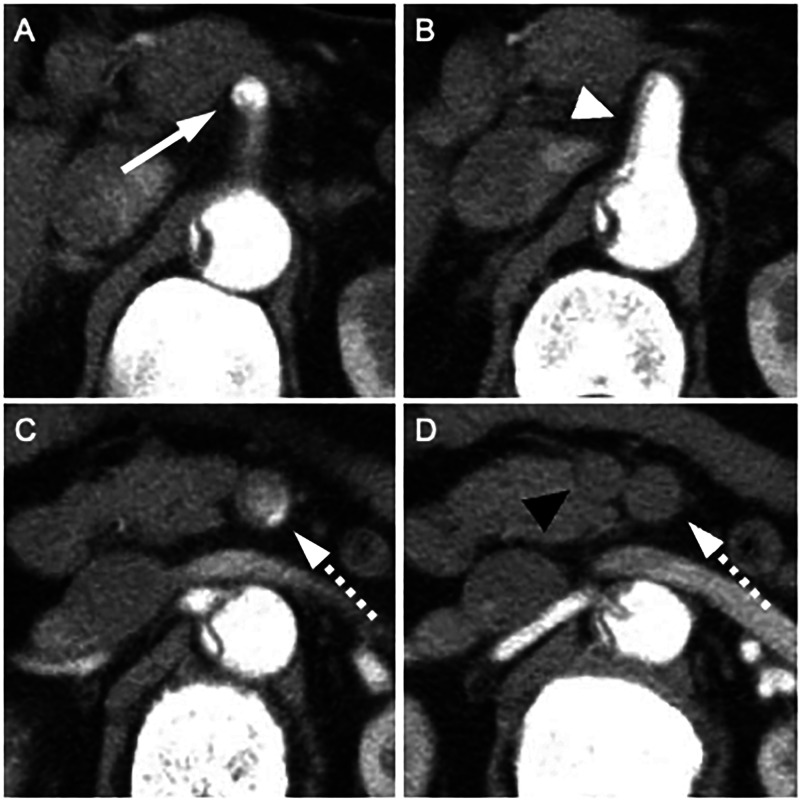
CT findings in patients with a celiacomesenteric trunk. (A) The celiac artery branch (white arrow) shows good contrast enhancement to the periphery. (B) The dissected celiacomesenteric trunk (white arrowhead) with a narrowed true lumen. (C) Superior mesenteric artery (SMA) branch (white dotted arrow) exhibiting poor contrast enhancement. (D) Peripheral occlusion of the SMA and presence of the superior mesenteric vein sign, characterized by a superior mesenteric vein diameter (black arrowhead) smaller than the SMA diameter (white dotted arrow), indicative of mesenteric ischemia.
